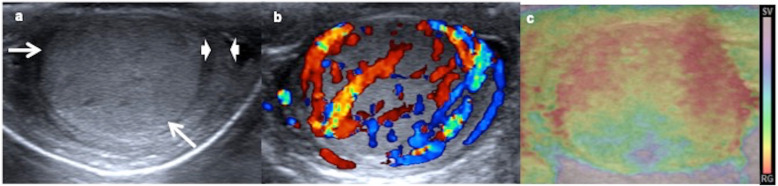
Yolk sac tumour. A 15-month-old boy with a painless swollen left testicle. a Longitudinal view of the left testis shows near-complete replacement by a hypoechoic solid mass (arrows) with a rim of normal parenchyma (arrowheads). b Power Doppler reveals increased blood flow within the tumour. c Elastography shows heterogeneous with high strain of the mass. The boy has a very high level of alphaphetoprotein and inguinal orchiectomy is practised. Yolk sac tumour is the definitive pathologic diagnosis
